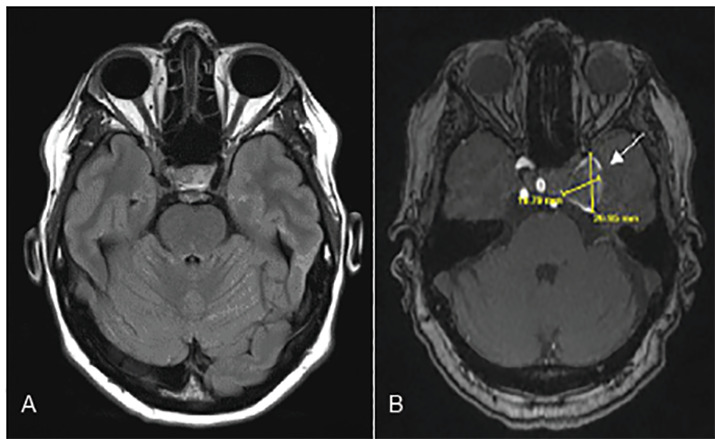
Internal carotid artery aneurysm. (A) Normal brain magnetic resonance imaging (MRI) (image courtesy: Prof. Frank Gaillard, Radiopedia.org) (B) Patient’s MRI showing thrombosed internal carotid artery aneurysm.
